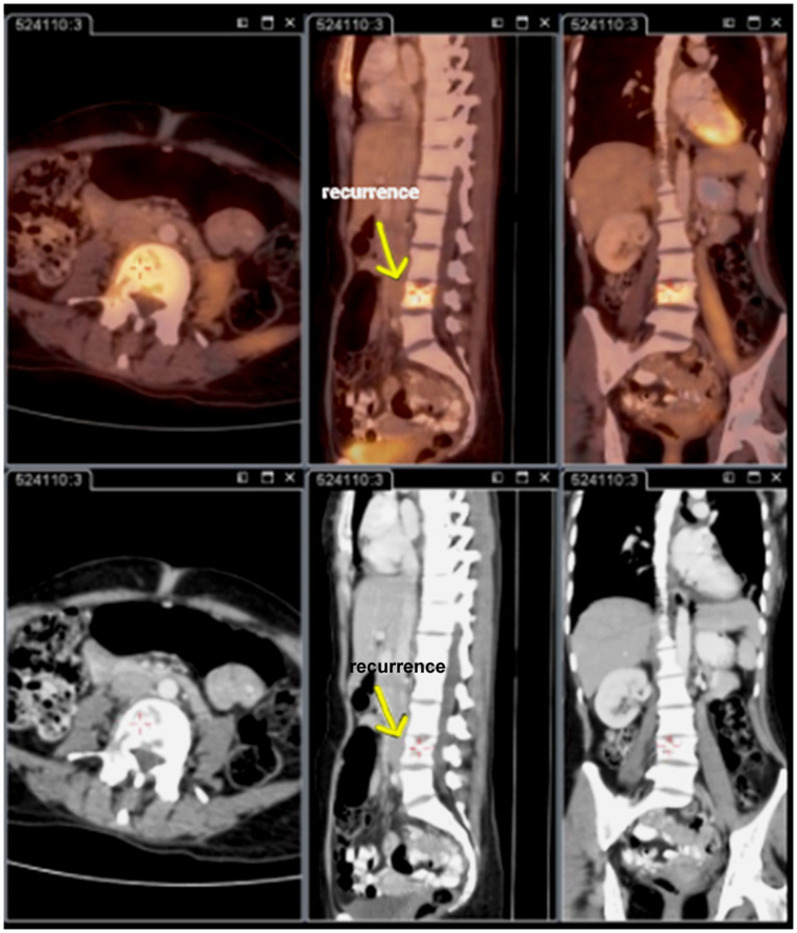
Whole body PET-CT performed for isolated CA 15-3 elevation (45-year-old female, 2-years post treatment) revealed an FDG avid
